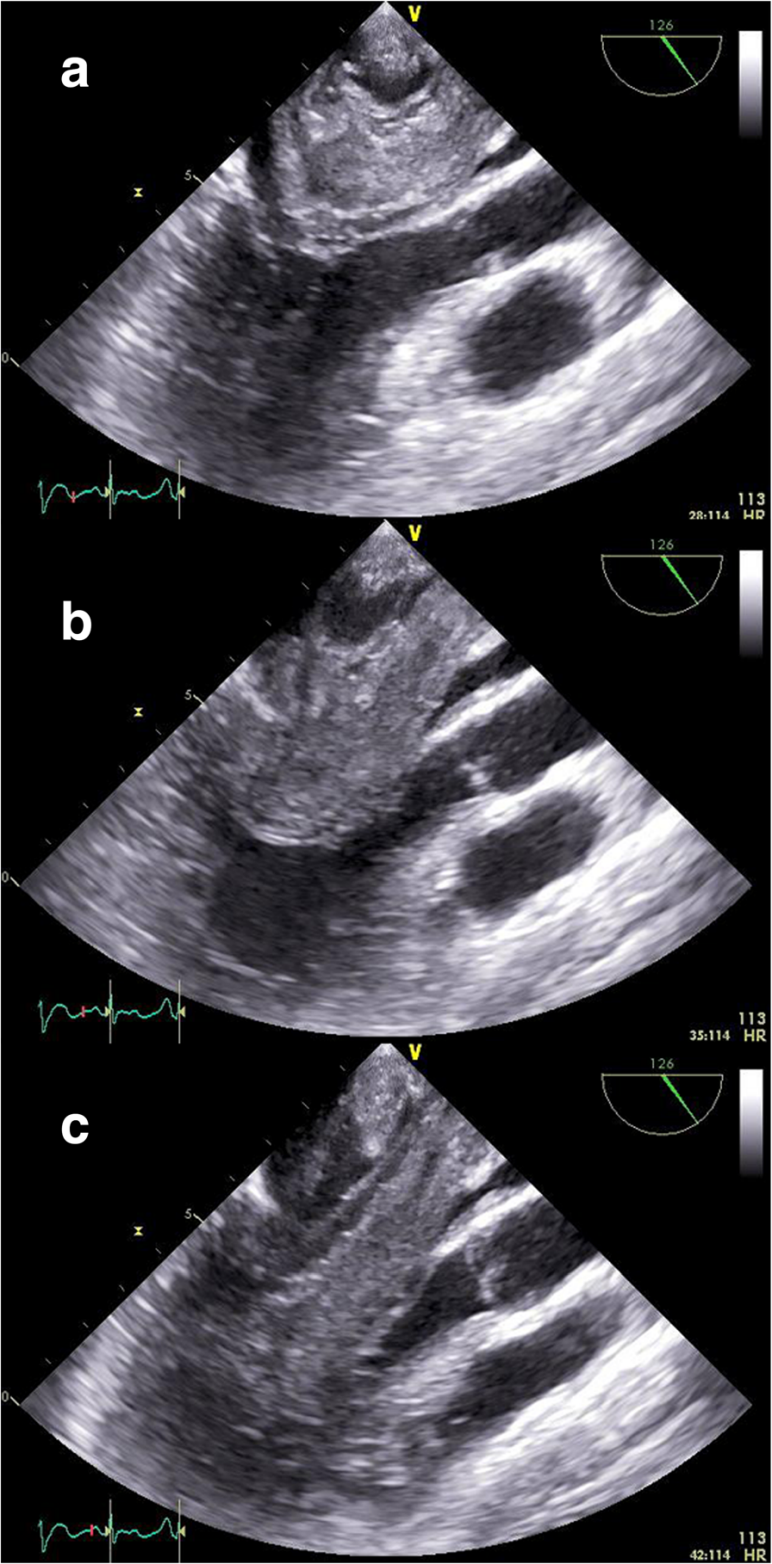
Pre-operative trans-esophageal echocardiogram images. The mobile tumor contracted inside the left atrium during a systolic phrase (a) and expanded across the mitral valve (b) into the left ventricular during a diastolic phrase (c)
